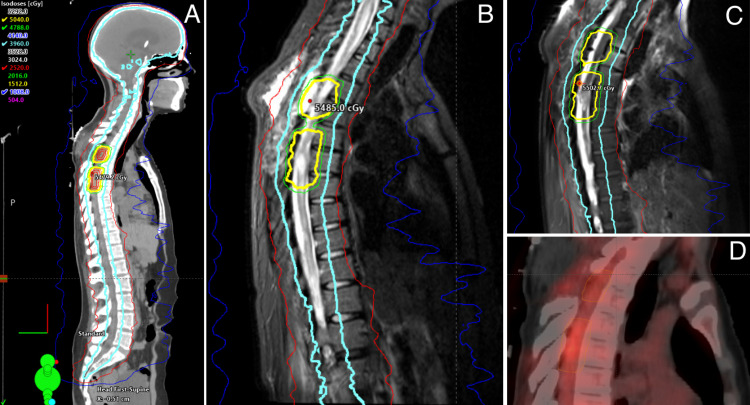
(A) Treatment planning CT (sagittal view) displaying total craniospinal dose of 39.6 Gy with sequential boost dose to a total of 50.4 Gy. (B) Sagittal STIR MRI displaying 50.4 Gy dose. (C) T1 sagittal post-contrast MRI displaying 50.4 Gy dose. (D) PET/CT (sagittal view) displaying increased PET avidity in the spinal cord tumor CT, Computed tomography; STIR, short-TI inversion recovery; MRI, magnetic resonance imaging; PET, positron emission tomography.
